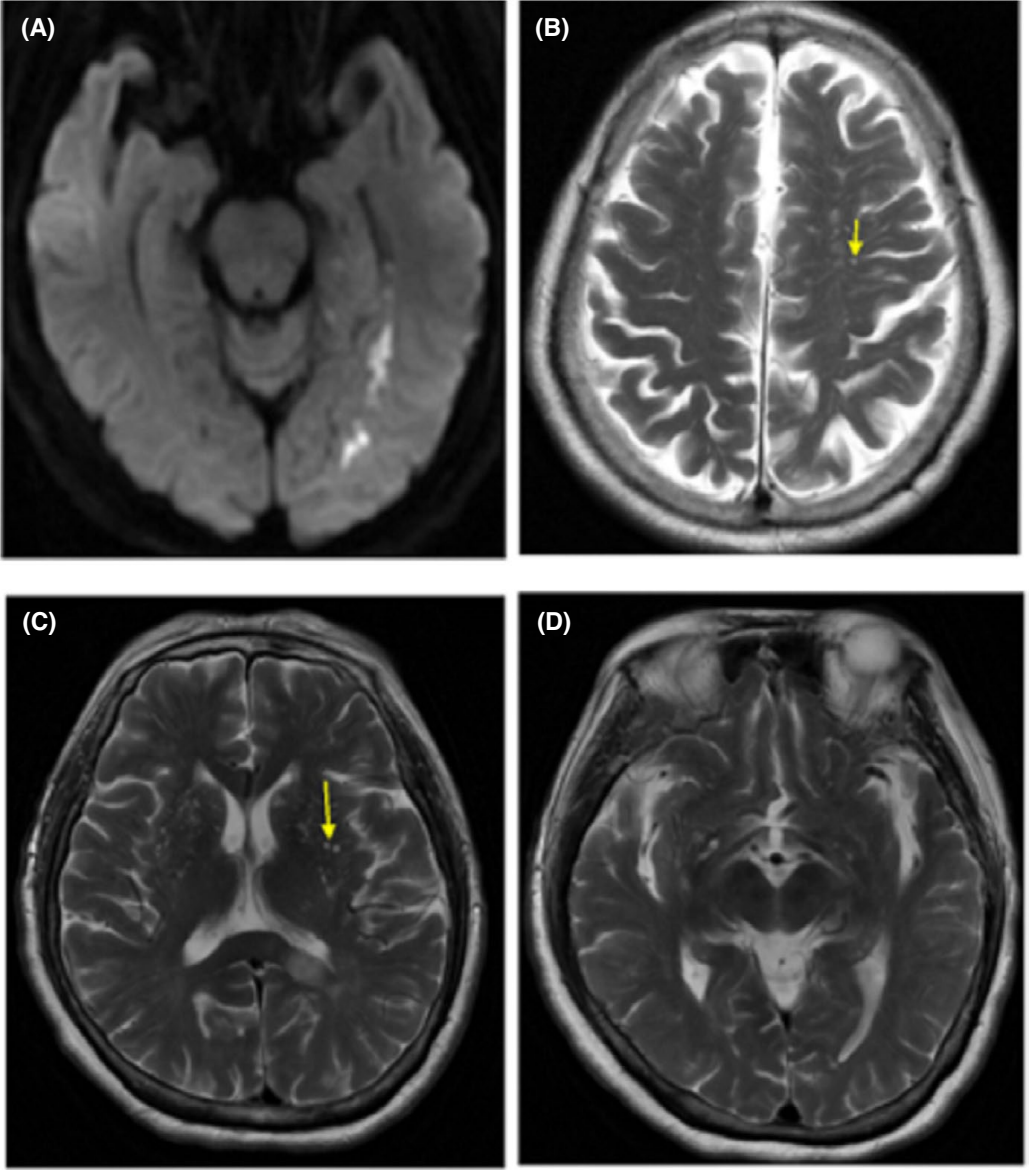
An example of PVS distribution in a patient with post‐stroke epilepsy. This was a 65‐year‐old woman presenting with retarded response to callings. She experienced an unprovoked seizure 7 months later. (A) Axial DWI showed acute infarction of left mesial temporal lobe. (B) Axial T2 showed a significantly asymmetric distribution of PVS in CS with more in left side. A similar effect was not observed in BG (C) and MB (D). BG, basal ganglia; CS, centrum semiovale; MB, midbrain; PSE, post‐stroke epilepsy
